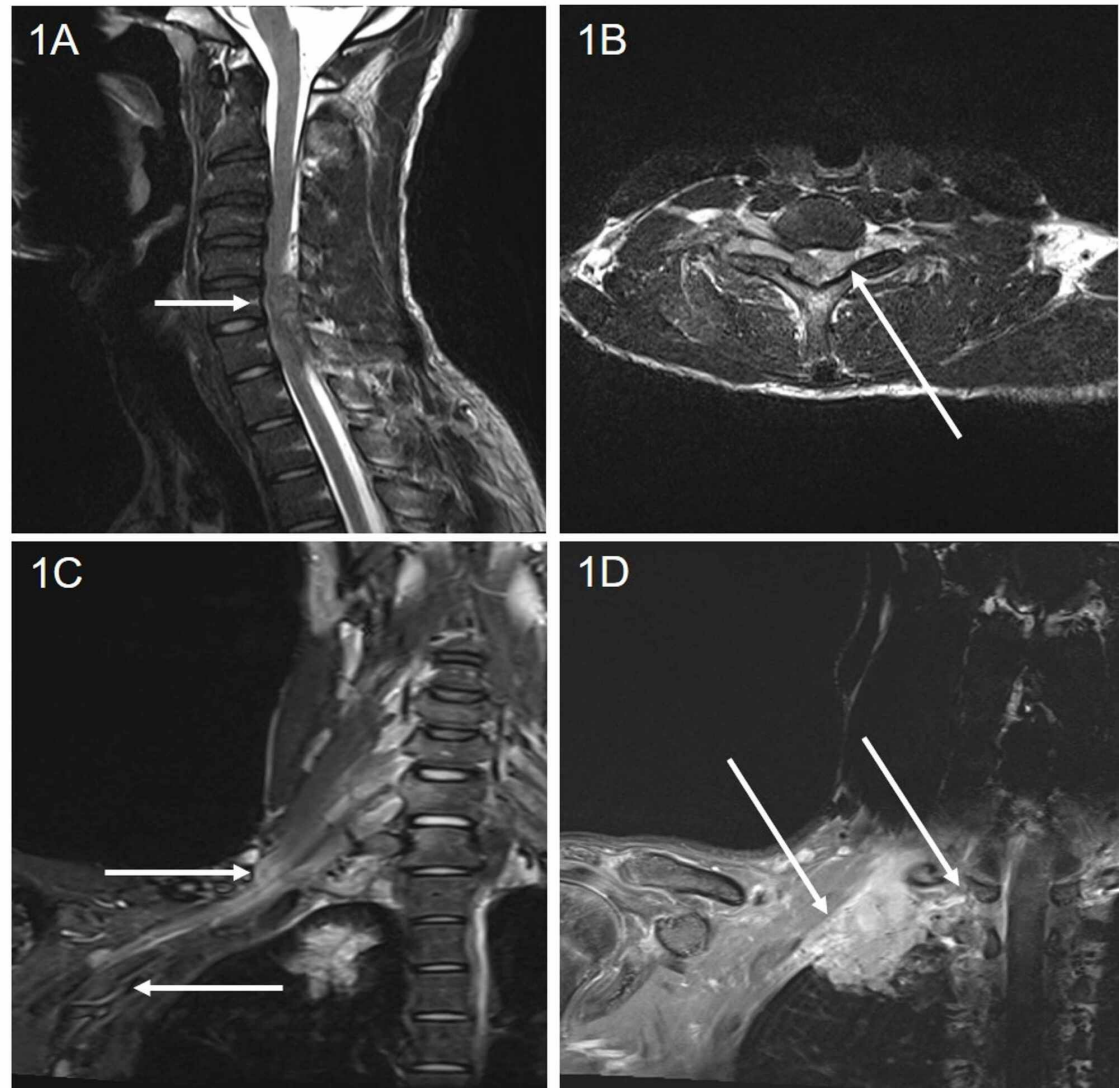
MRI images of cervical spine and right brachial plexus (1A) MRI of the cervical spine - sagittal view of the neck showing large heterogeneous enhancing right-sided extradural mass in the spinal canal extending over a distance of 9 cm and also extending into all right neural foramina from C3-C4 through C7-T1 levels. (1B) MRI of the cervical spine - axial view again showing extradural mass displacing the spinal cord to the left with evidence for osseous remodeling along the mass. (1C) MRI of the right brachial plexus showing thickened and hyperintense trunks, divisions and cords of the right brachial plexus, which probably represent neuritis. (1D) MRI of the right brachial plexus showing abnormal signal in the right transverse processes of C6 and C7 vertebra, and right aspects of C7 and T1 vertebral bodies, consistent with bone involvement.
